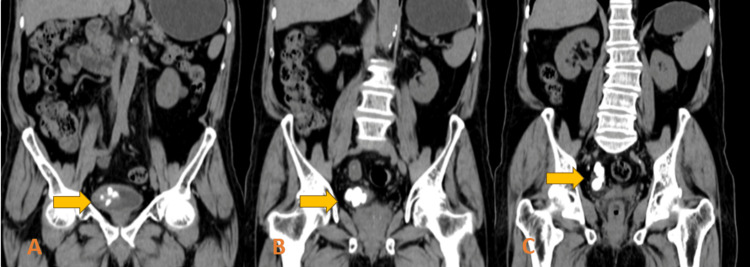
Coronal on-contrast CT abdomen showing multiple hyperdense calculi in the invaginated part of the right ureter in the urinary bladder i.e. in the ureterocele (A); in the vesico-ureteric junction (B); and in the distal ureter (C)
